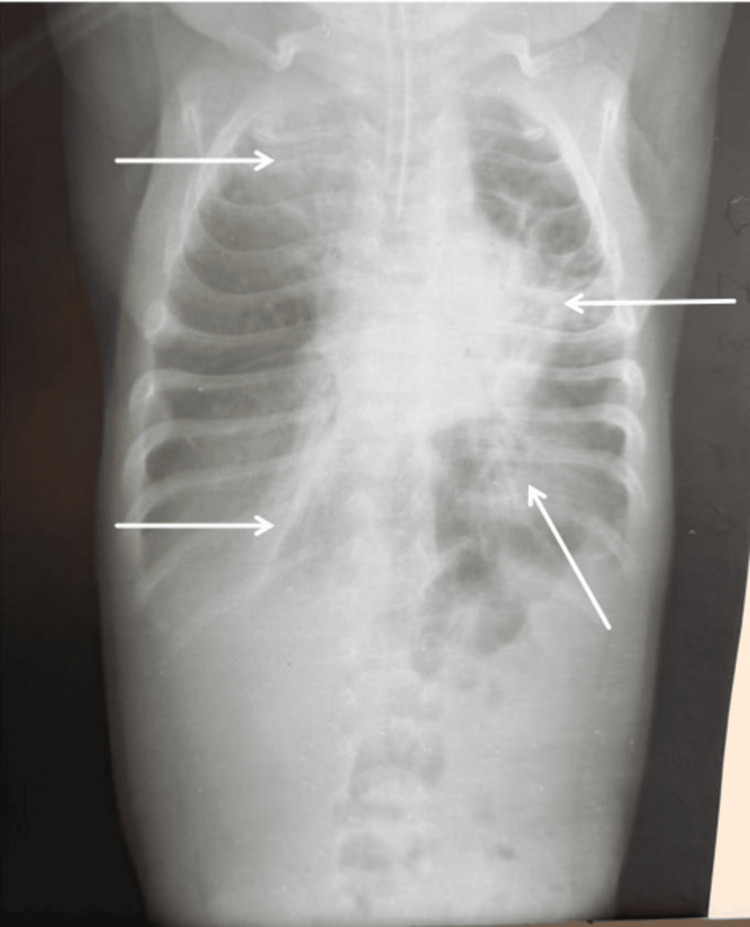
Chest radiograph demonstrating bilateral infiltrates at admission Arrows indicate areas of consolidation consistent with pneumonia.
